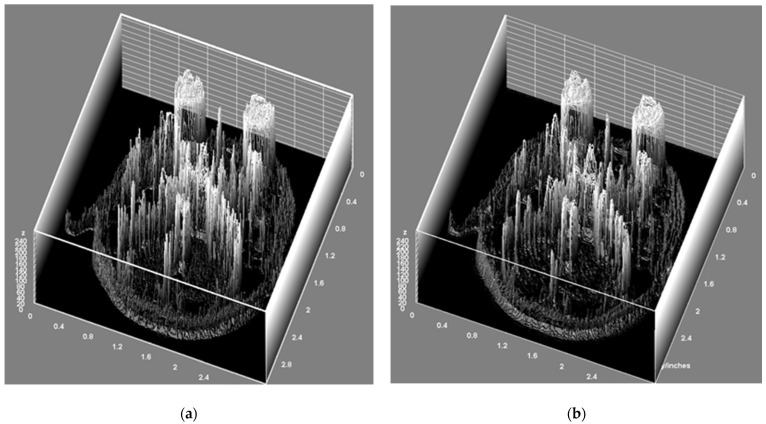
This Figure shows the texture components of the MRI data reconstructed using SR compared with those reconstructed using GT. (a) Represents the GT and (b) displays a high-resolution image based on the SR. This comparison suggests that there were no significant changes in texture composition when the MRI data were reconstructed using SR.
